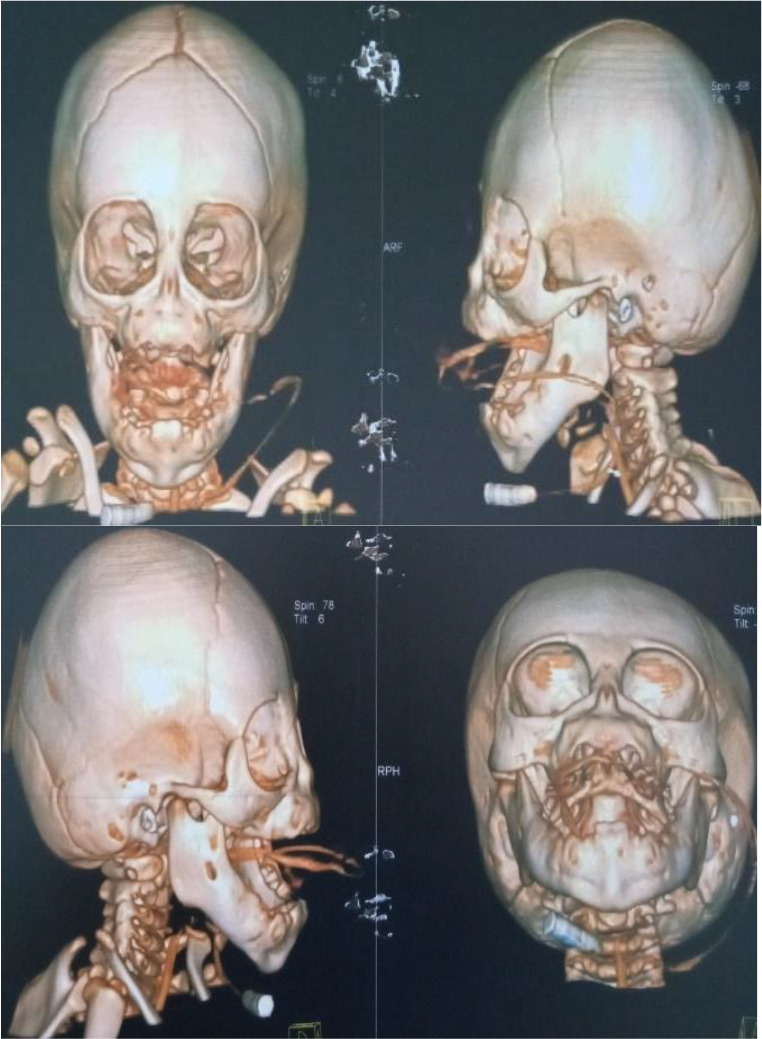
A 3D Head and upper airway CT scan showing complete absence of the nasal bone, internal nasal structures and complete stenosis of the nasal canals.
